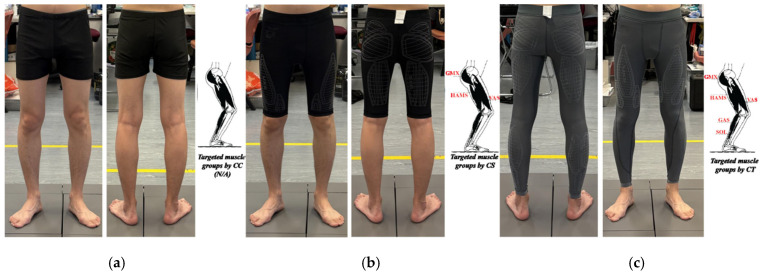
Three types of legwear: (a) CC, (b) CS, and (c) CT.
